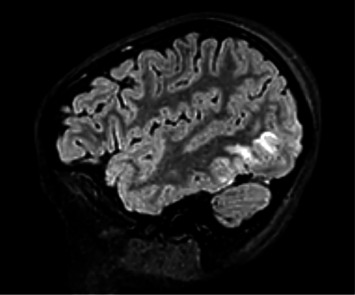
Sagittal MRI T2-FLAIR sequence displaying vertical hyperintensities in the posterior aspect of the left temporal lobe.
